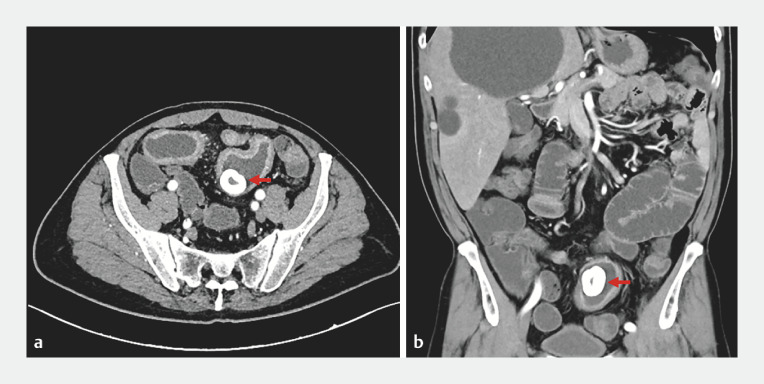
Computed tomography revealed distal small intestinal wall thickening with a 3.4 × 2.8 cm high-density intraluminal foreign body (red arrow).
